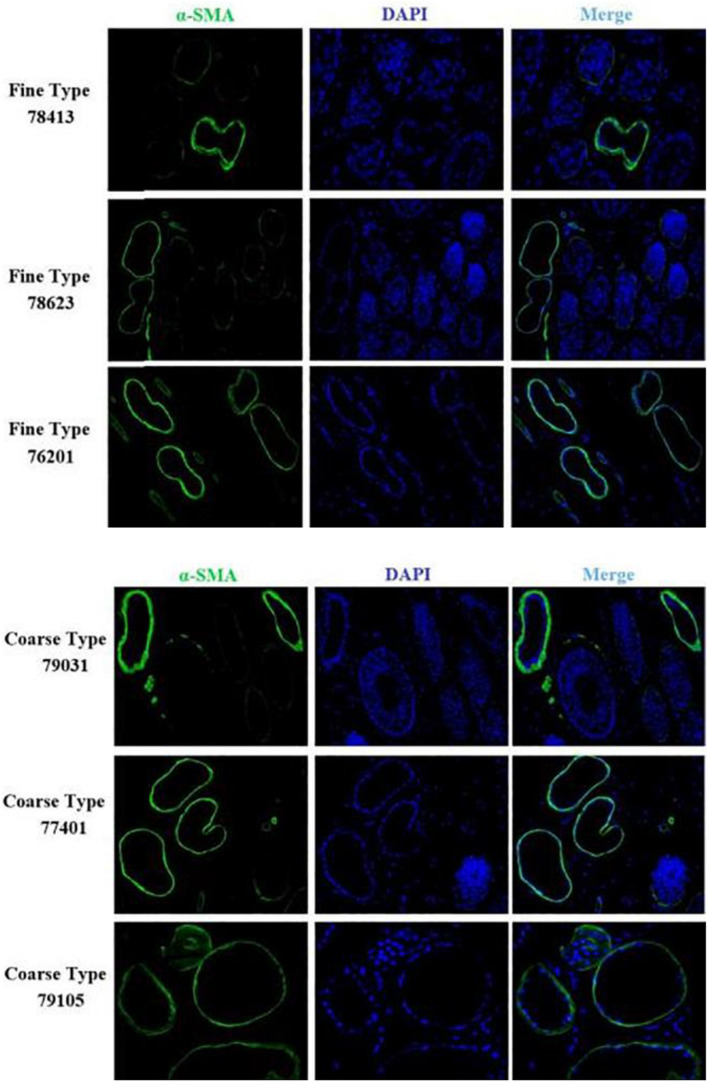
IF results of *ACTA2* (*α-SMA*) in FT-LCG and CT-LCG skin tissues (200x). *ACTA2* is basically expressed in SHFs.
